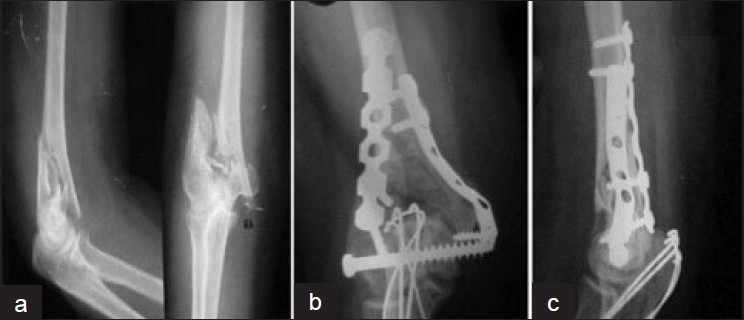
Radiograph (a) showing C2 type fracture stabilized by intercondylar cancellous screw and bicolumnar orthogonal fixation (b) by contoured plates. Extra-articular osteotomy (c) is stabilized by two parallel K-wires and tension band wire
